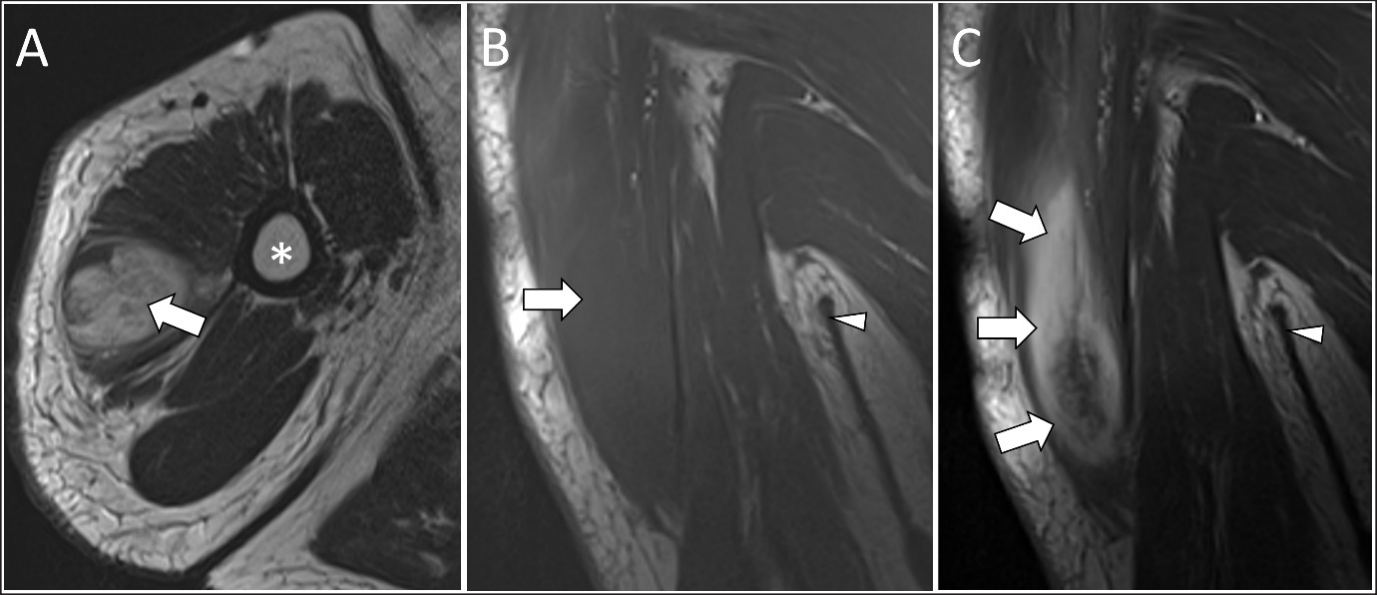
MRI images of PM in the right deltoid muscle demonstrate a hyperintense lesion (white arrow) on axial T2WI (A) and an isointense lesion on coronal T1WI (B) with a marked enhancement on coronal post-contrast T1WI (C) (white arrows). Humerus (*). Axilla (white arrowhead).
